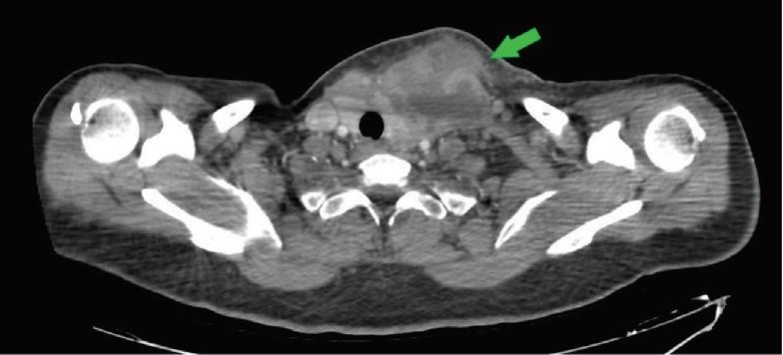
CT scan shows a fluid collection with a thick, irregular wall in the lower cervical region. Tracheal deviation is seen.
